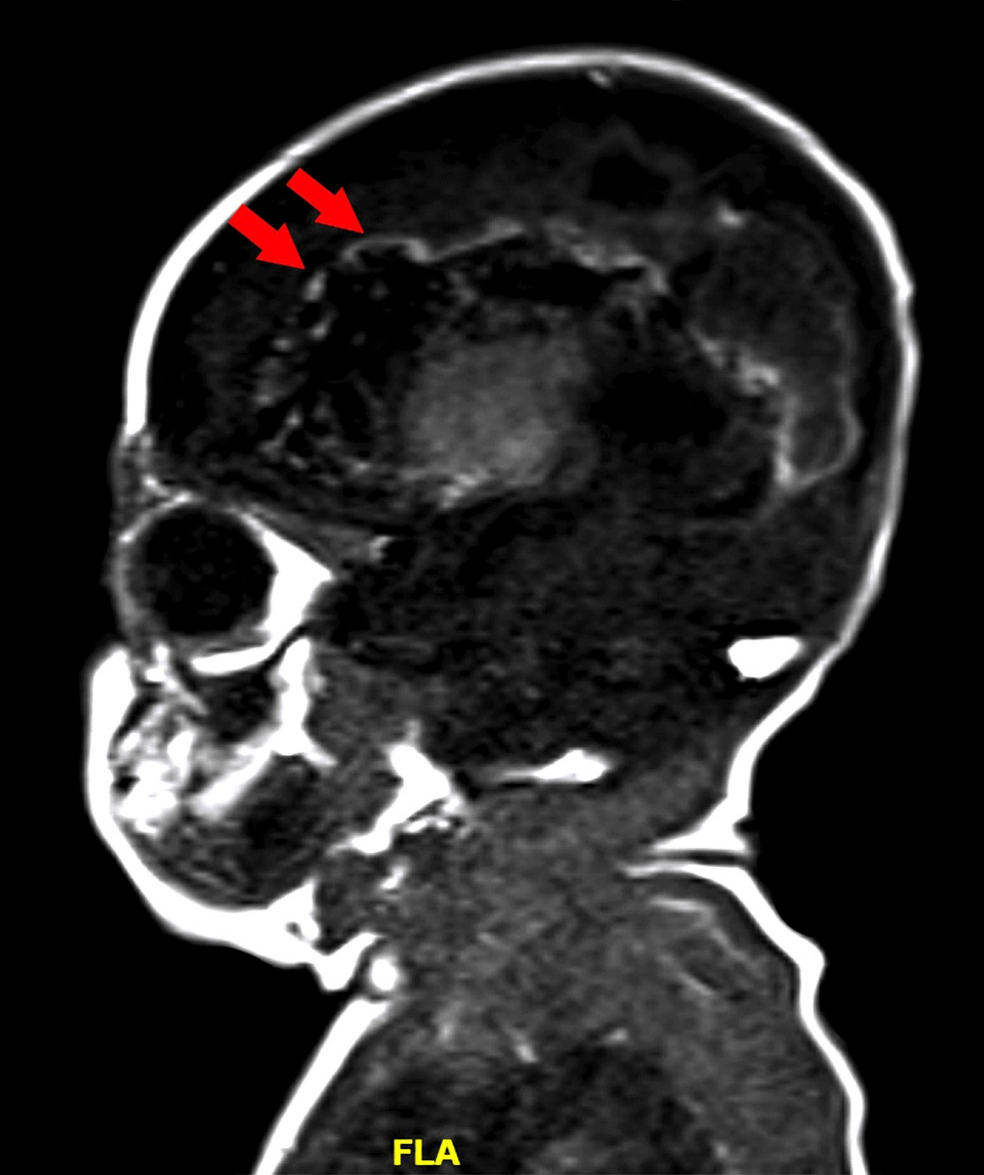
T1-weighted MRI in the sagittal plane demonstrating bilateral cystic foci, primarily in the distribution of the right MCA on postoperative day 21. MCA: middle cerebral artery
